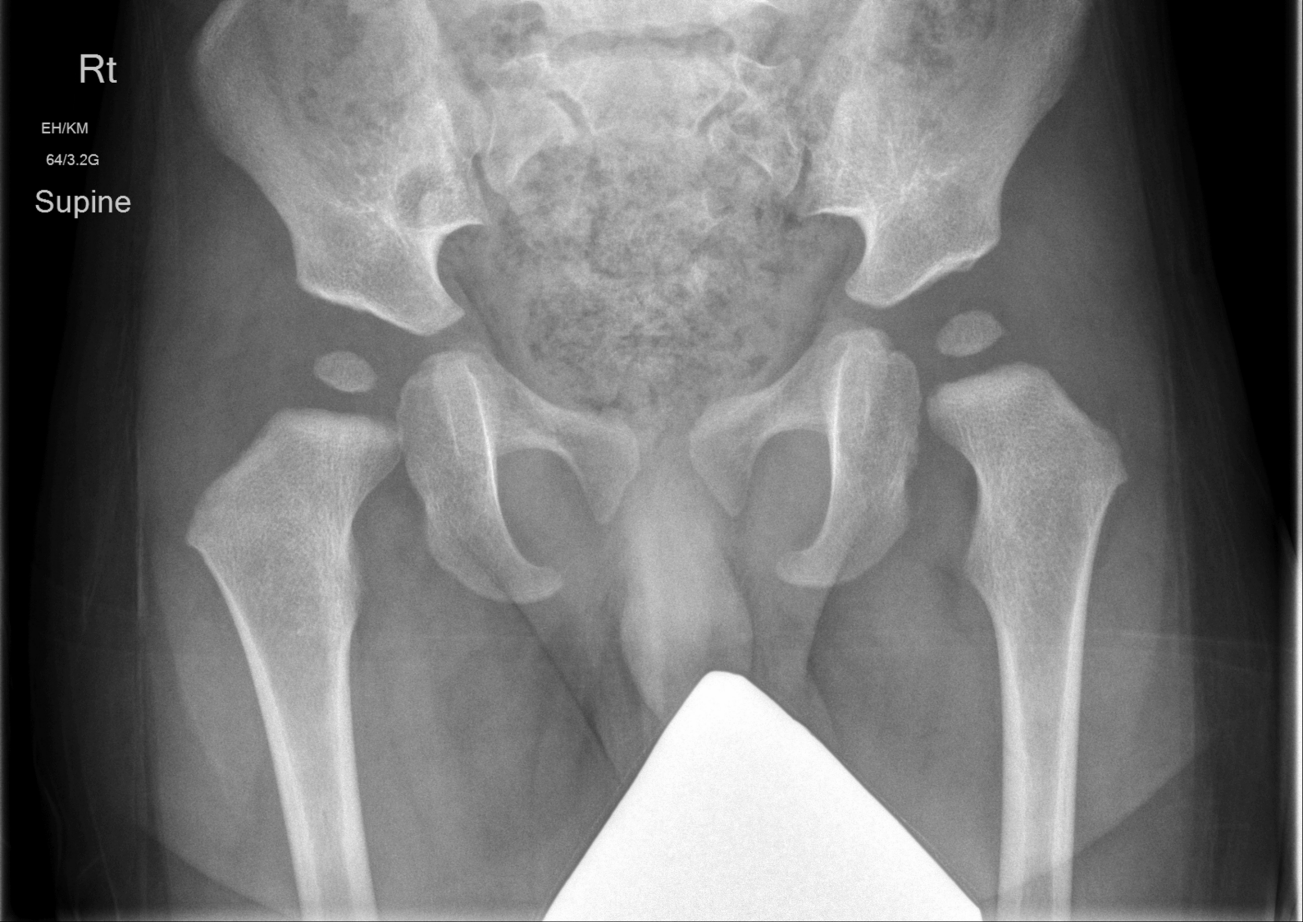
Pre-intervention pelvic X-ray. Acetabular index was 27.5° on the right and 28.4° on the left. Rt: right
